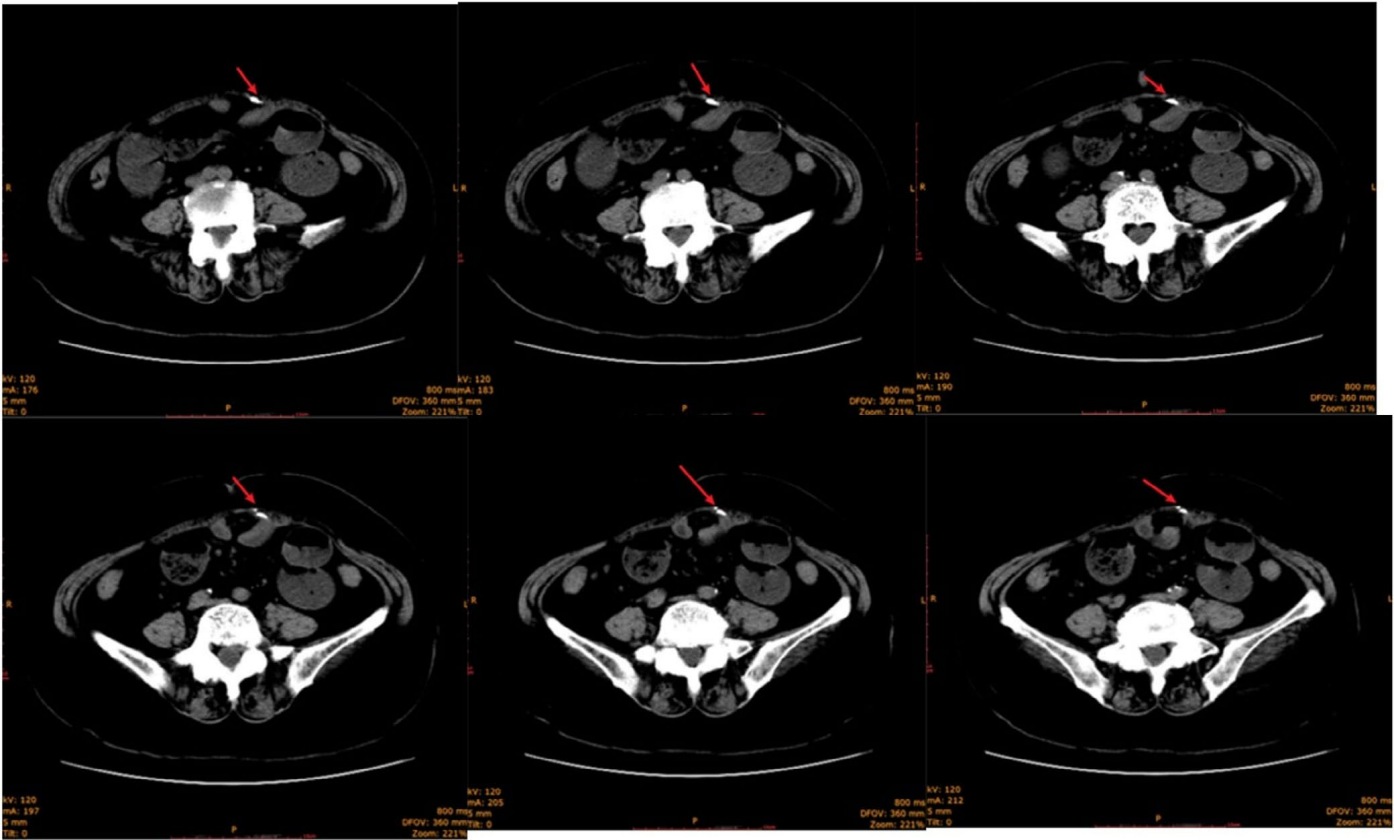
CT image showing an irregular mass embedded between the peritoneum and the posterior sheath of the rectus abdominis at the site of adhesion. The mass is well demarcated from the surrounding tissues but exhibits mild adhesion to the mesenteric vessels, resulting in angulation of the intestinal loop and proximal small bowel dilation.
